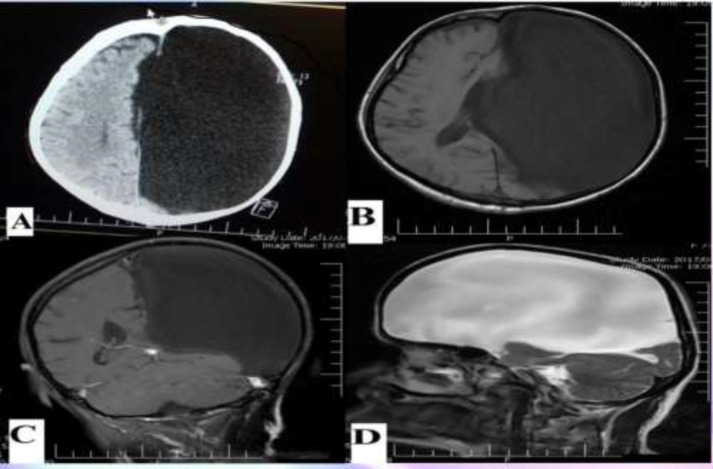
Axial non-contrast-CT scan showing a huge hypodense area in the left hemisphere of the brain
